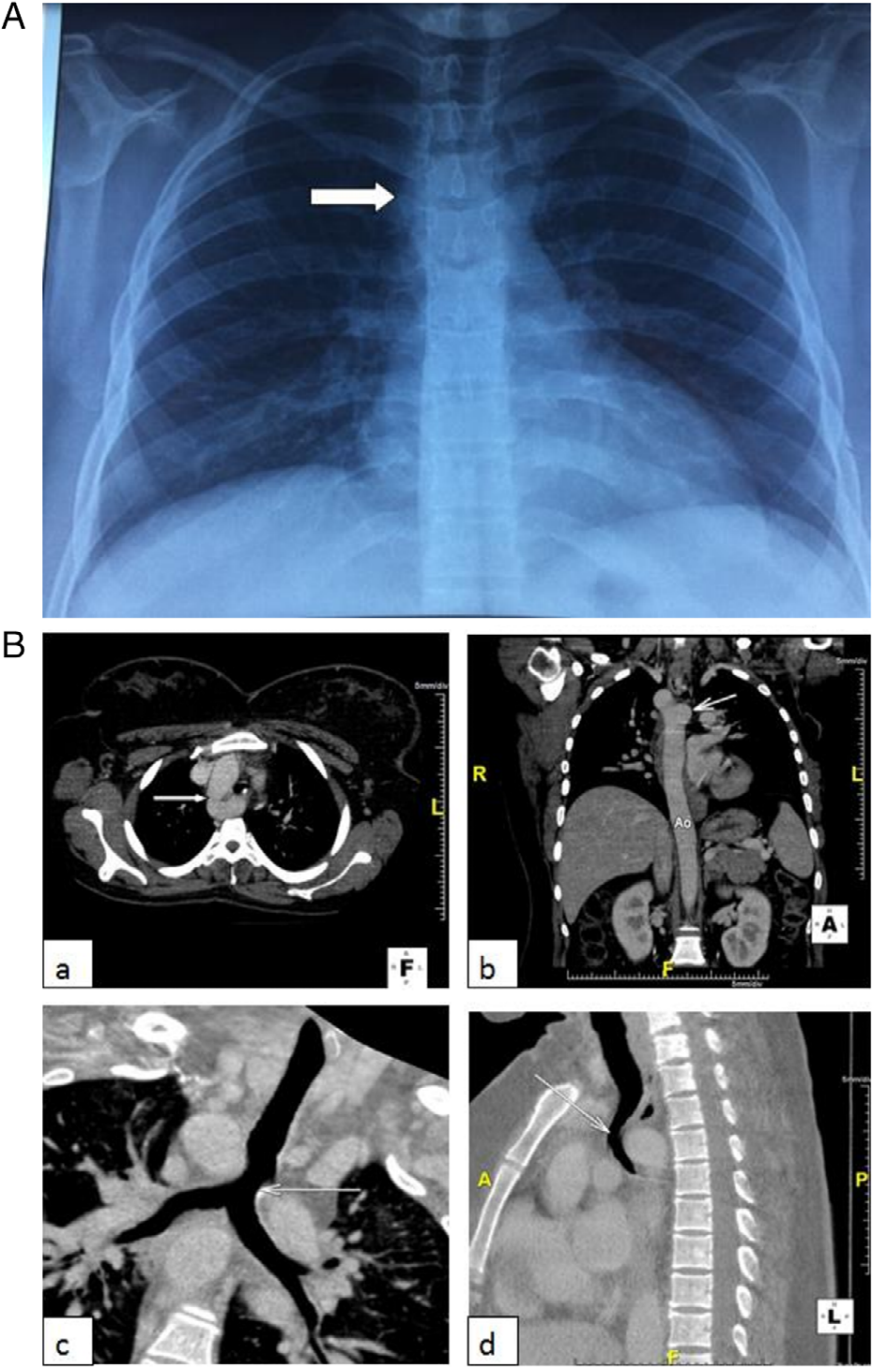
Imaging findings: (A) Frontal chest radiograph: right‐sided aortic arch with a right‐sided aortic knob (white arrow). (B) Chest CT Scan: a right‐sided aortic arch and a tracheal compression due to the diverticulum of Kommerell. (a) Axial image: right‐sided aortic arch (white arrow). (b) Coronal image: diverticulum of Kommerell (white arrow). (c) Coronal image (reconstruction): tracheal compression (white arrow). (d) Sagittal image: tracheal compression (white arrow).
